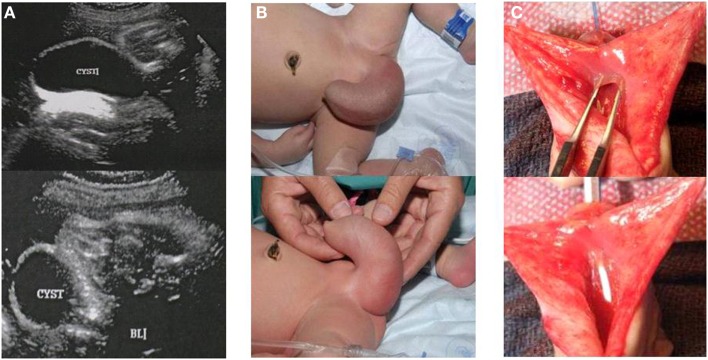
Male patient with anterior urethral diverticulum. (A) Prenatal ultrasound showing large cystic mass in the genital area. (B) Enlarged urethral bulging mass, megalourethra. (C) Picture depicting anterior urethral valve during open urethroplasty.
